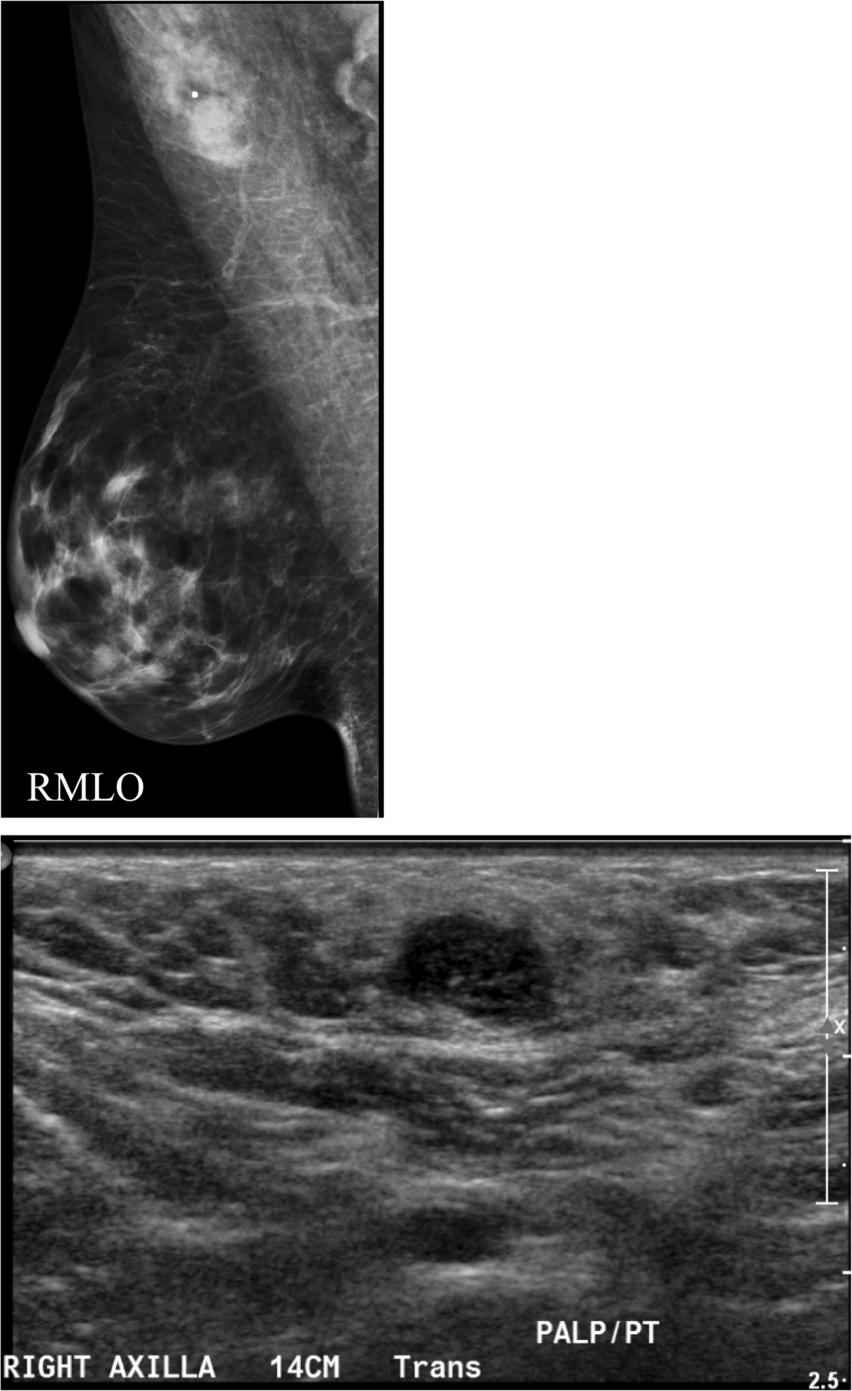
Accessory breast tissue with fibroadenoma: a BB placed on the skin in the area of concern to the patient. MLO views show hetereogeneously dense 4 cm asymmetry of similar density and morphology to breast tissue. b Targeted sonographic evaluation of the right axilla in the area of concern demonstrates fibroglandular breast tissue and a hypoechoic 8-mm solid mass. Biopsy of the nodule using a no-throw vacuum-assisted biopsy device revealed fibroadenoma
